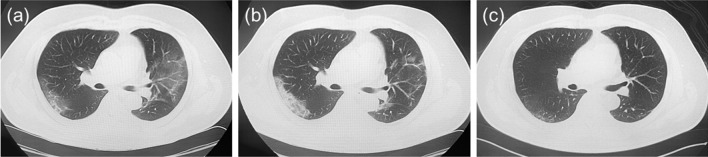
Chest computed tomography (CT) images. a CT image 5 days after onset of symptoms. Bilateral ground-glass opacities (GGOs) were observed with lower-lung predominance. b CT image taken 11 days after onset, showing slightly elevated CT values of GGOs. c CT image taken 54 days after onset, showing the disappearance of GGOs
